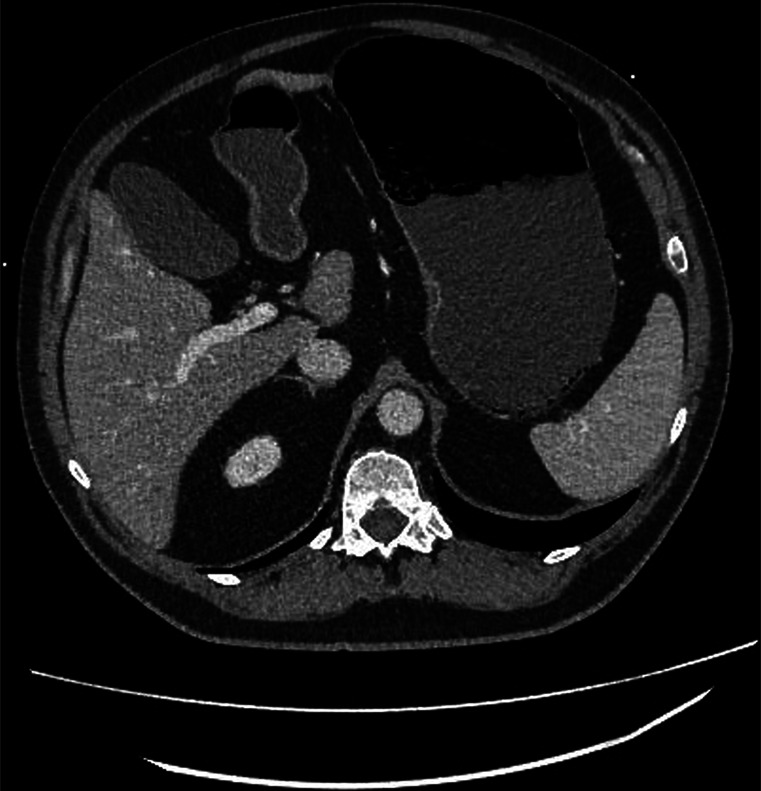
Computed tomography of the abdomen showing gastric pneumatosis in the gastric cardia and proximal fundus.
